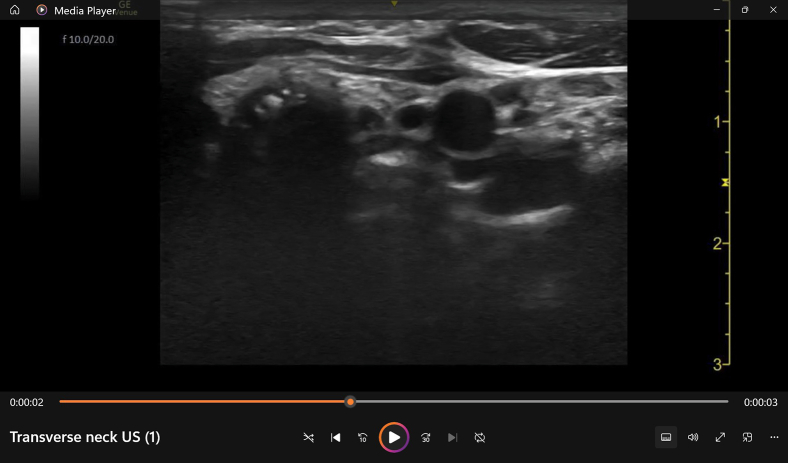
Transverse neck ultrasound demonstrating a hyperechoic foreign body with posterior acoustic shadowing within the distal esophagus, posterior to the trachea.
